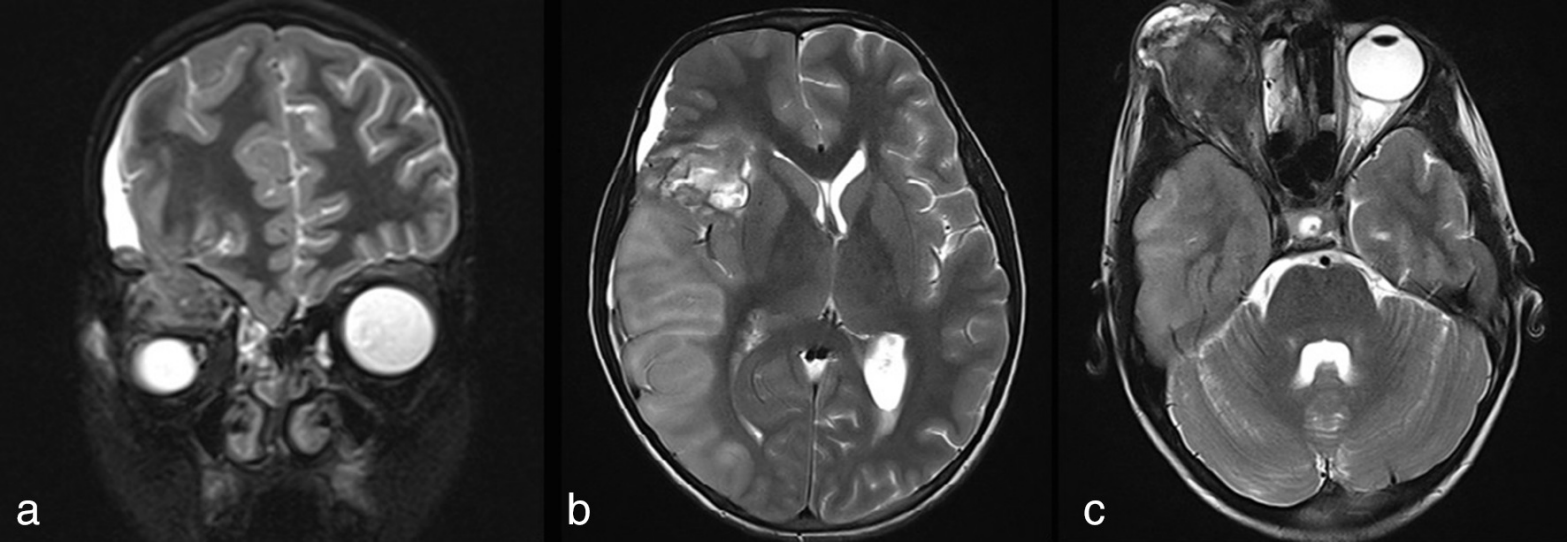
Coronal thin section (a) and axial and sagittal (b, c) *T*_2_ weighted MRI showing herniation of the brain tissue from the cranial cavity into the right orbit. Contusion injury is seen in the right frontal lobe with associated oedema and midline shift towards the contralateral side. Proptosis is seen in the axial image.
